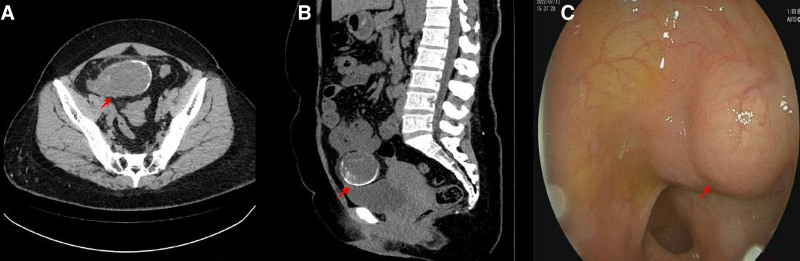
Contrast-enhanced computed tomography (CT) and endoscopic images in low-grade appendiceal mucinous neoplasm (LAMN). (A) Axial view of the enhanced CT image displays an eggplant-like swelling in the appendix with heterogeneous low density and patchy enhancement within the lumen. (B) Sagittal CT image depicts cystic dilation of the appendix featuring a widened root, enhanced wall, and calcifications reminiscent of eggshells. (C) Colonoscopy image reveals normal mucosa, a spherical impression in the submucosal layer, displacement of the appendiceal orifice, and soft tissue intercepted by biopsy forceps (indicated by arrows).
